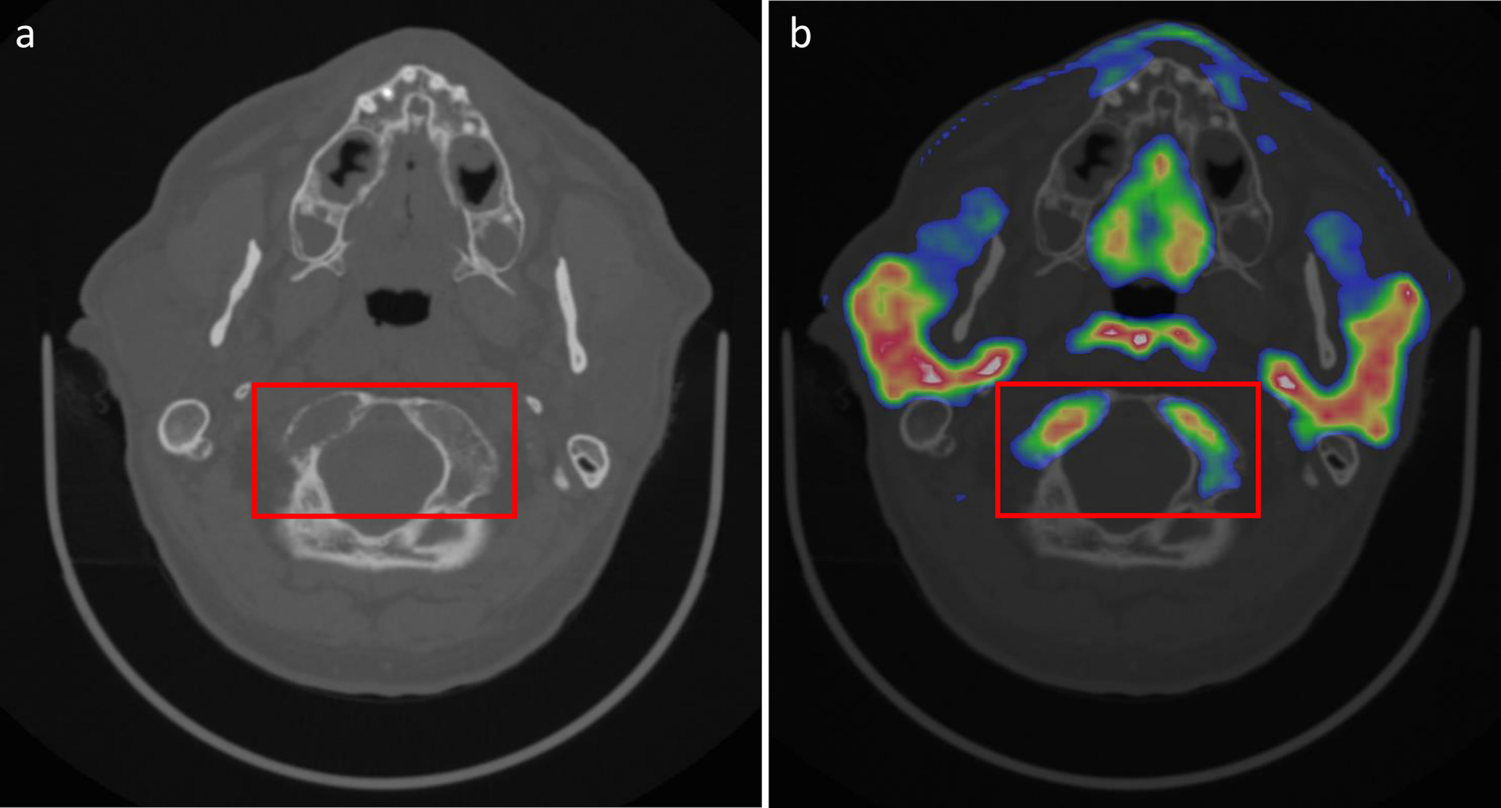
(a) Bony invasion of tumour (indicated by the red box), as evidenced by disrupted trabecular meshwork pattern observed on bone window CT scan, and (b) confirmed on axial ^11^carbon methionine PET-CT scan. Normal PET tracer uptake is seen in the parotid glands, posterior wall of the nasopharynx and the mucosa of the hard palate. Bone marrow uptake in uninvolved bone was not marked.
